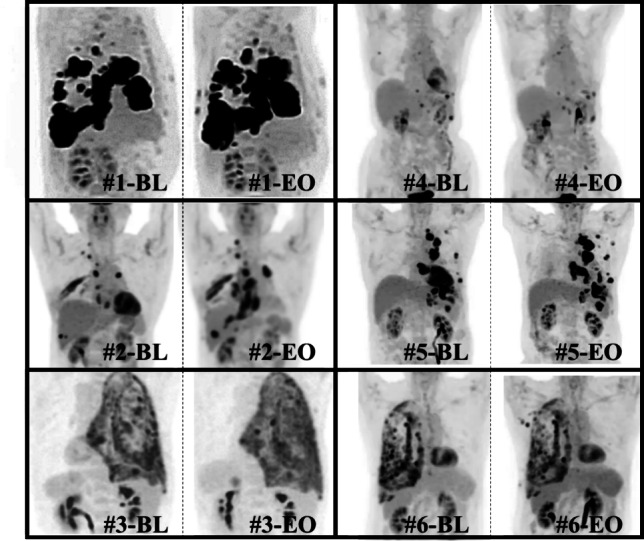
Whole tumor imaging using positron emission tomography (PET)/computed tomography (CT)
